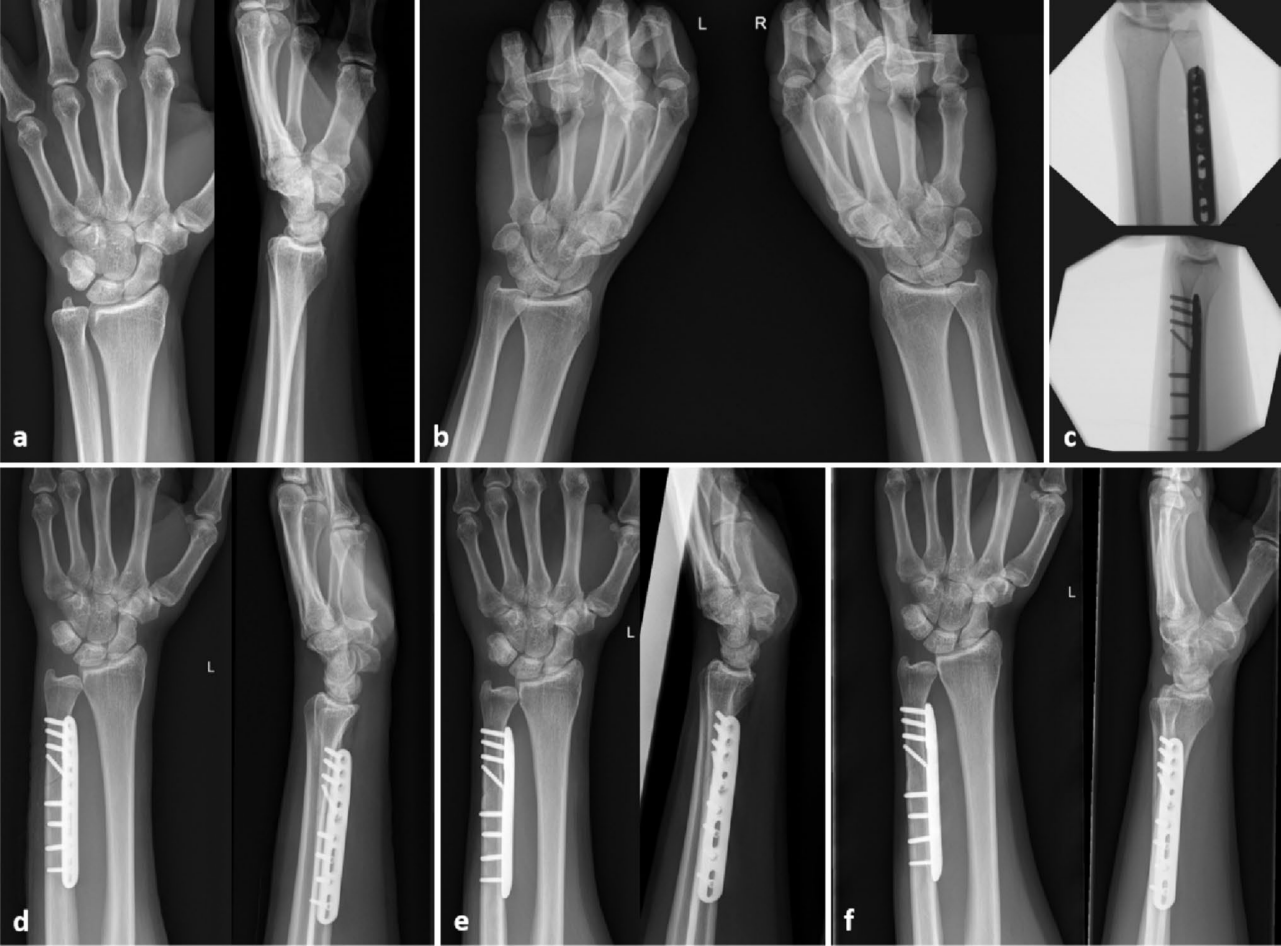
Case with a dynamic ulnar impaction syndrome successfully treated with the UOL Plate II. a X-ray preoperatively, b clenched fist X-ray preoperatively showing a dynamic positive ulnar variance on both sides, c intraoperative fluoroscopic control, d–f postoperative X-ray two weeks, three and six months postoperativelyshows an example of a case with a left sided dynamic ulnar impaction syndrome treated with the I.T.S. UOL System II
